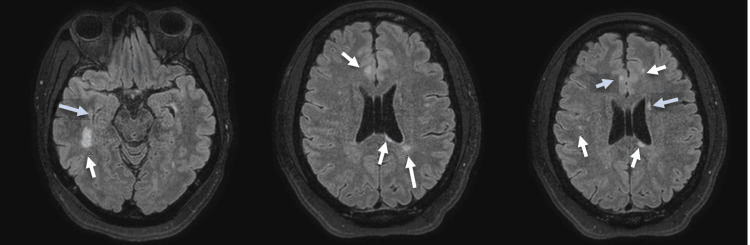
FLAIR magnetic resonance images of the head. Multifocal evolving FLAIR signal abnormality lesions over time when compared to previous MRIs five months ago with interval enhancement of the subependymal lesion in the posterior superior margin of the body of the left lateral ventricle at its junction with the left ventricular atrium, concerning for demyelinating disease such as seen in multiple sclerosis.
